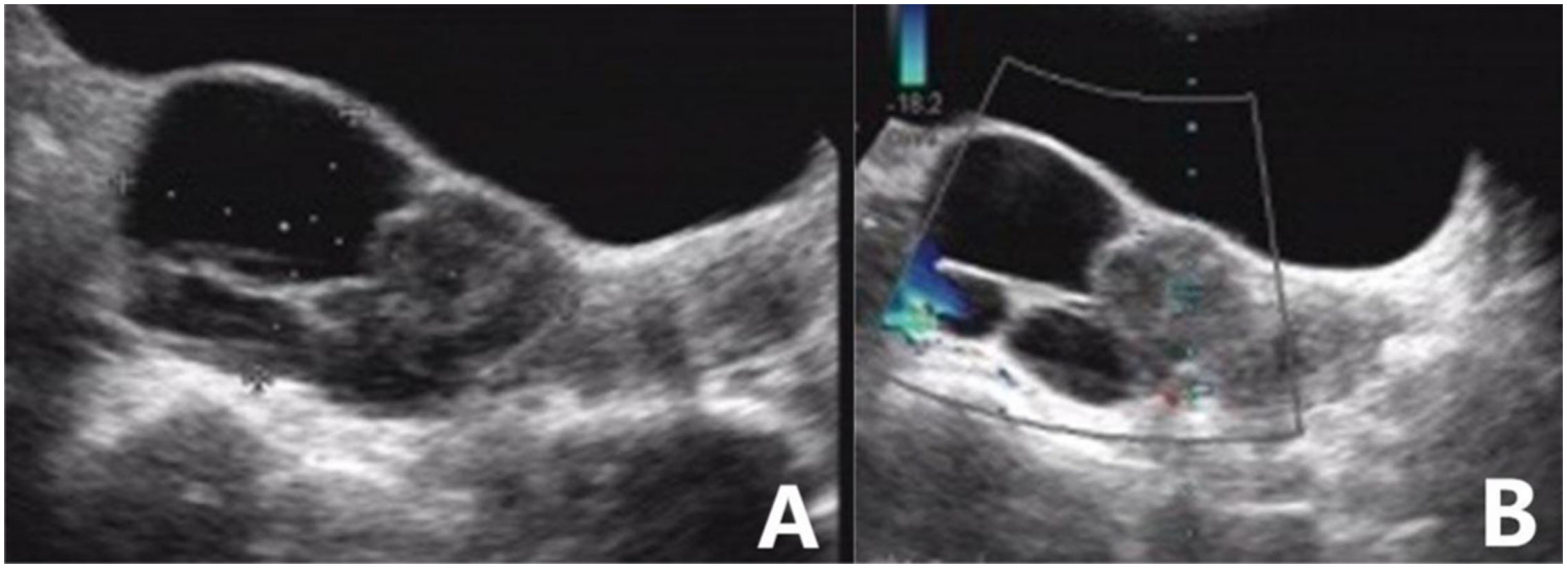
High-grade serous carcinoma of the ovary. (A) Sonography revealed a mixed echogenic mass in the posterior right of the uterus, with well-circumscribed cysts, a solid half, and internal multilocular septa. (B) A small amount of blood flow signal could be detected in the solid component of the mass. The blood flow parameters were as follows: PSV, 12.5 cm/s; EDV, 4.2 cm/s; and RI, 0.67. The GI-RADS classification was 4a, and the tumor was considered to be malignant. The risk value of the ADNEX model was 57.8%; for malignant tumors, the pathological type was predicted to be stage II-IV epithelial ovarian cancer. Postoperative pathological results revealed high-grade serous carcinoma.
